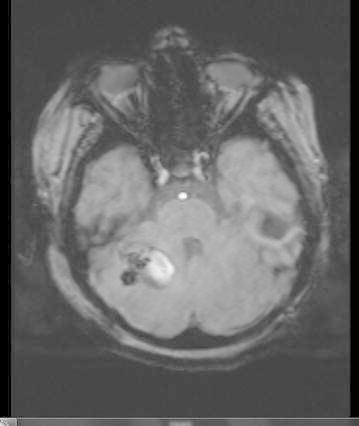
Postoperative cranial MRI showed a regressed lesion
